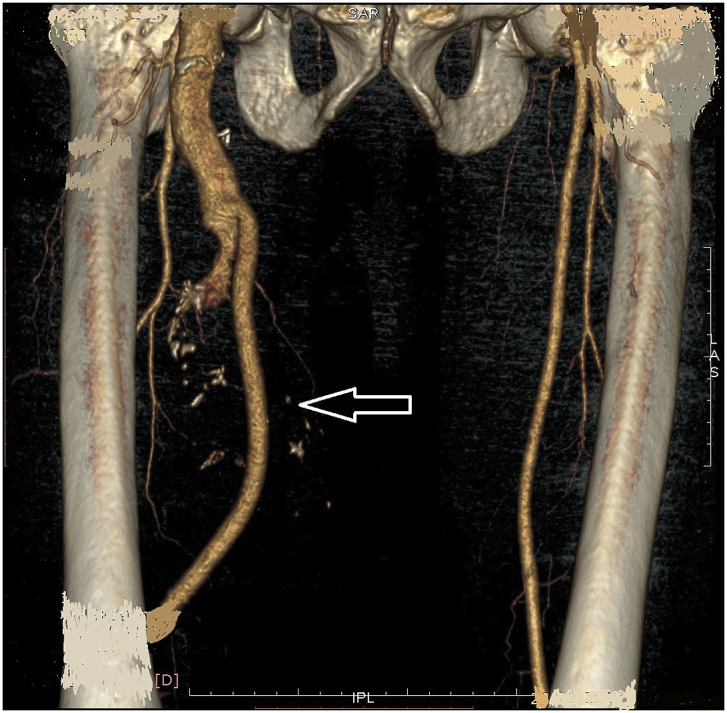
CTA post-operative,arrow show new anastomosis graft.
